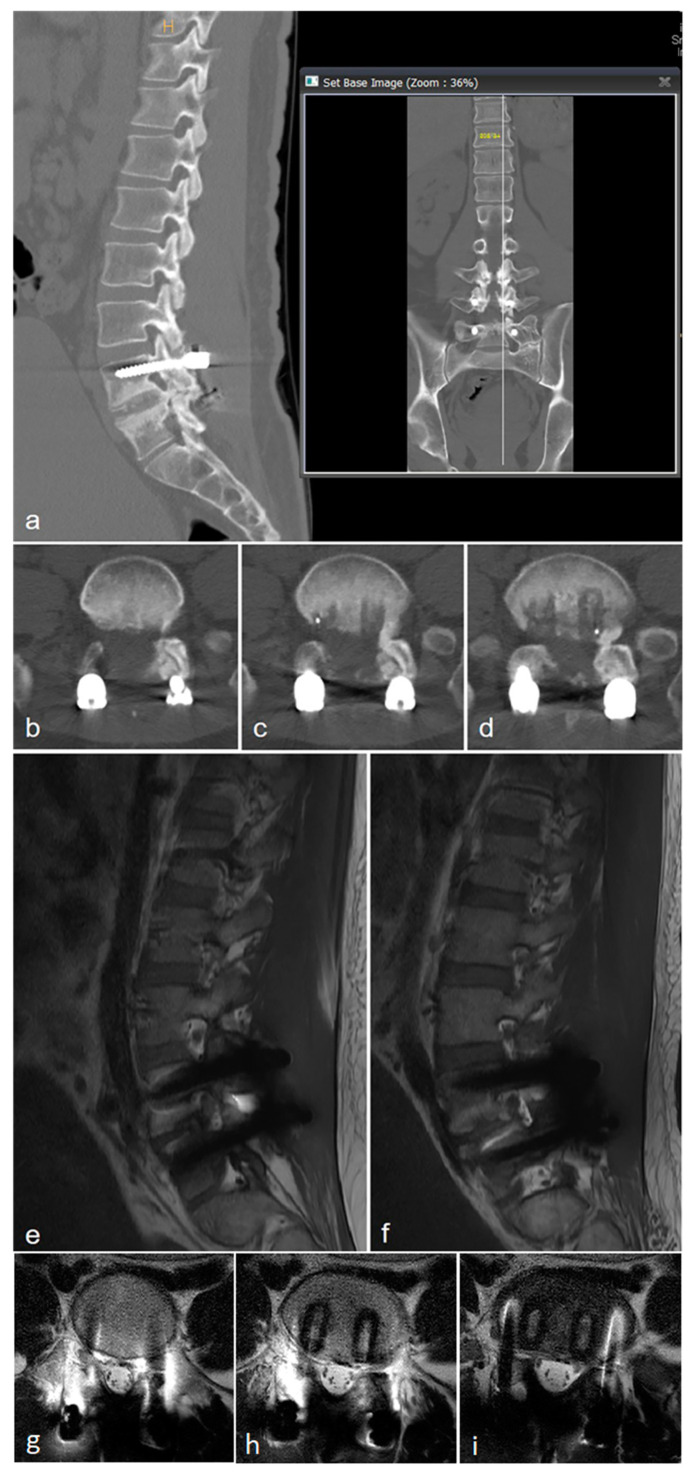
Images 5 years after the surgery. (a–d) CT image shows restenosis of facet joint on left side at L4/L5 level. (e–i) L-spine MR image shows restenosis of left side at L4–5 level.
